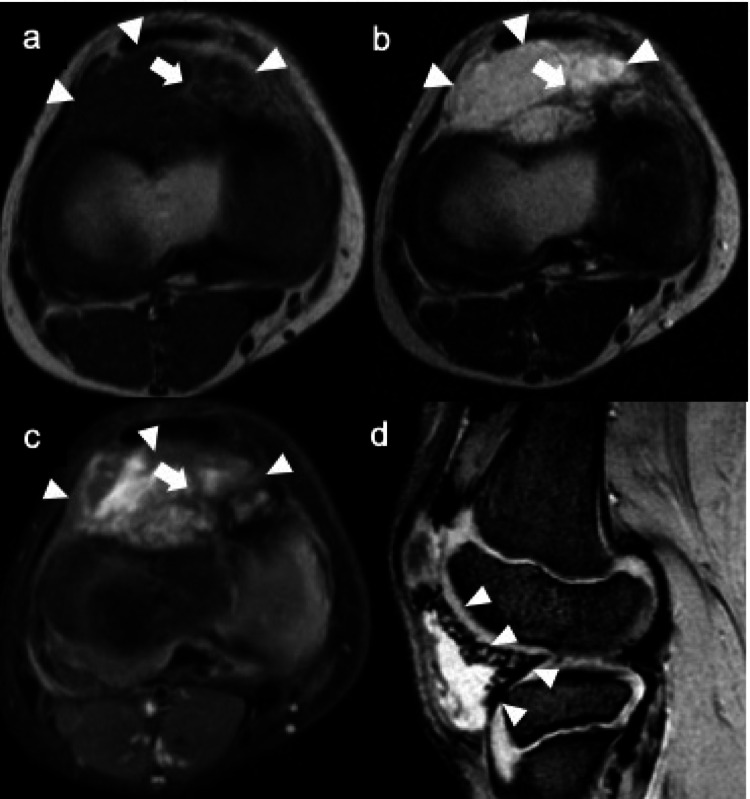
Synovial hemangioma. Axial T1-weighted MR image (a) shows heterogenous intensity mass (arrowheads) containing high signal areas (arrow) in Hoffa’s fat pad. On axial T2-weighted MR image (b), the mass is high signal intense to subcutaneous fat. Fat-suppressed postcontrast MR image (c) shows heterogenous enhancement. Sagittal T2-weighted MR image (d) shows a hyperintense mass with low signal intensity septa in Hoffa’s fat pad (arrowheads) and thick synovium with low signal intensity, representing hemosiderin deposits due to repeated intra-articular hemorrhage
